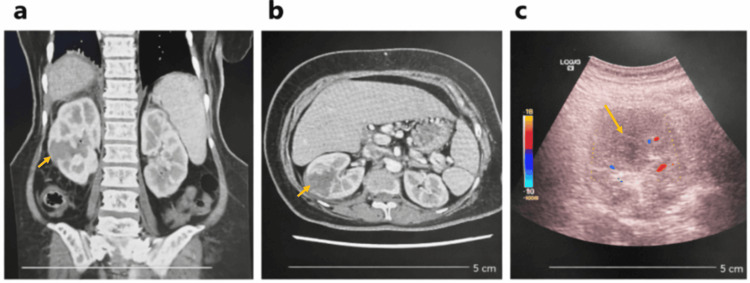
Imaging findings of the renal abscess. (A) Coronal contrast-enhanced CT image demonstrating a hypodense lesion in the kidney with features consistent with a renal abscess, associated with surrounding inflammatory changes. (B) Axial contrast-enhanced CT image showing the renal abscess with peripheral enhancement and adjacent perinephric fat stranding. (C) Grey-scale and color Doppler ultrasound image revealing a heterogeneous mixed cystic lesion with minimal internal vascularity, supporting the diagnosis of a renal abscess.
